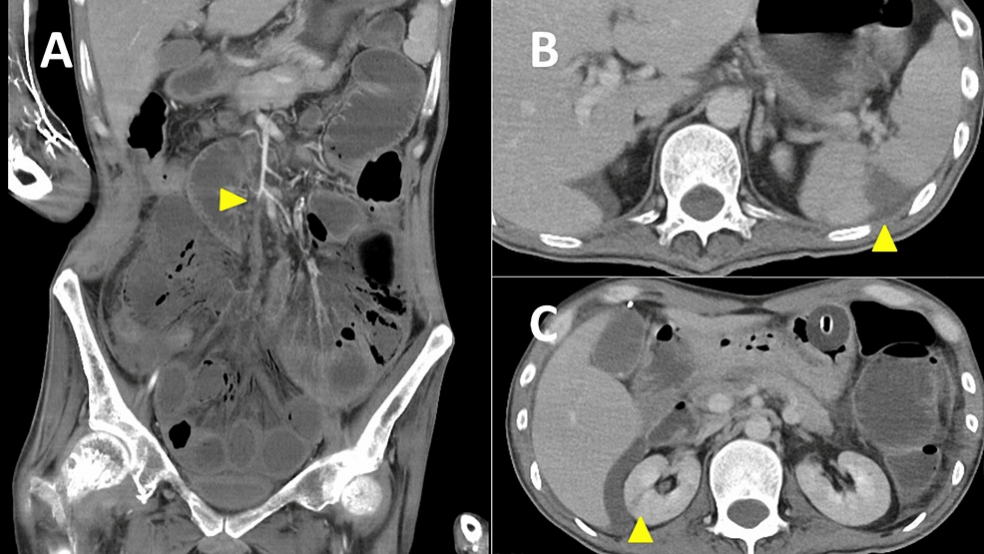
Contrast-enhanced computed tomography showing a superior mesenteric artery thrombus, ischemic colitis, ascites, and infarctions in the spleen and right kidney.
